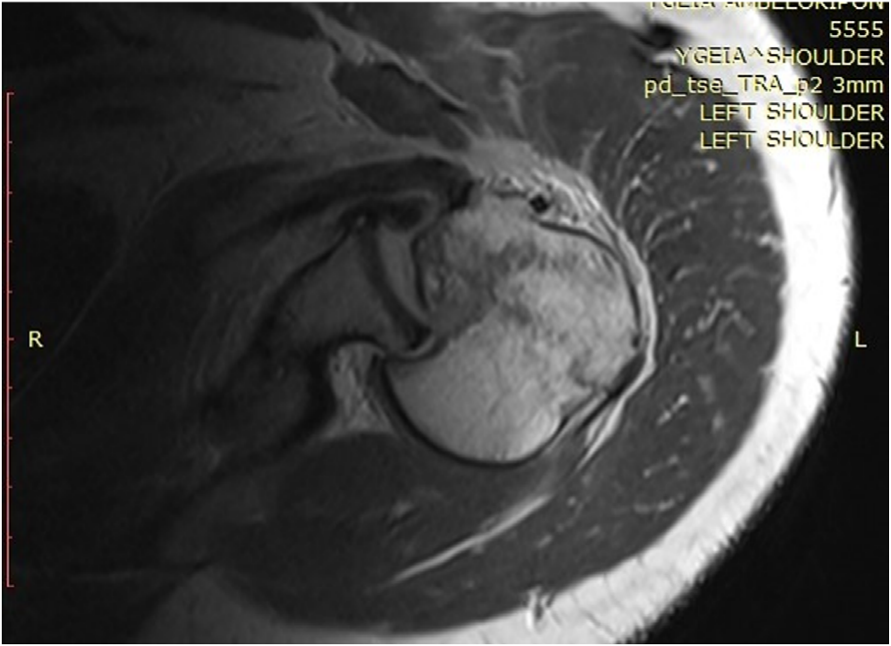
2nd patient: MRI scan showing PSD, reverse Hill-Sachs, minimally displaced humeral head fracture, reverse Bankart lesion, intact posterior glenoid rim, intact lesser tubercle, and subscapularis.
